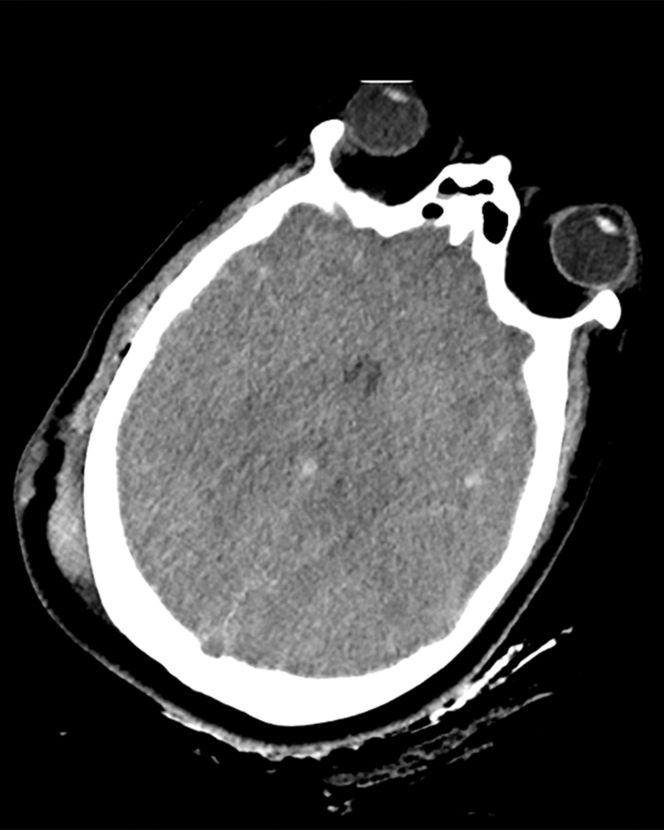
Axial head computed tomography: diffuse cerebral edema (hypodense parenchyma, effaced sulci) indicated traumatic brain injury.2.Axial abdominal CT: focal gas foci in the right hepatic vein confirmed hepatic venous pneumatosis, a core component of porto-mesenteric venous gas (PMVG). Isolated hepatic venous gas may be transient, but its coexistence with other abdominal findings elevated clinical suspicion (Fig 2).
